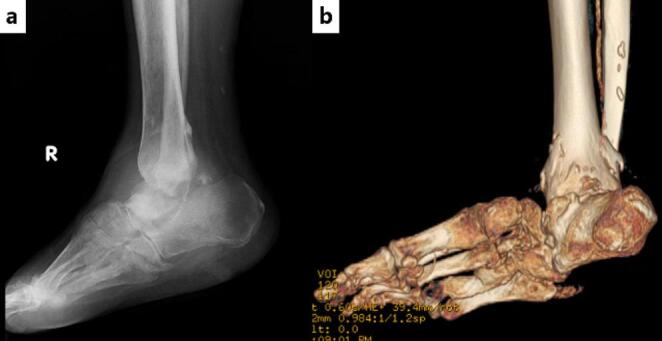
Charcot arthropathy. a lateral radiograph, b 3DCT. A woman in her 50s with type 2 DM presented with right foot deformity. Lateral radiograph (a) and CT (b) demonstrate marked deformity of the 3rd and 4th metatarsals, with multifocal periarticular osseous destruction and subchondral irregularity involving the calcaneus, navicular, cuboid, and cuneiform bones. Abbreviations: CT, computed tomography; DM, diabetes mellitus
